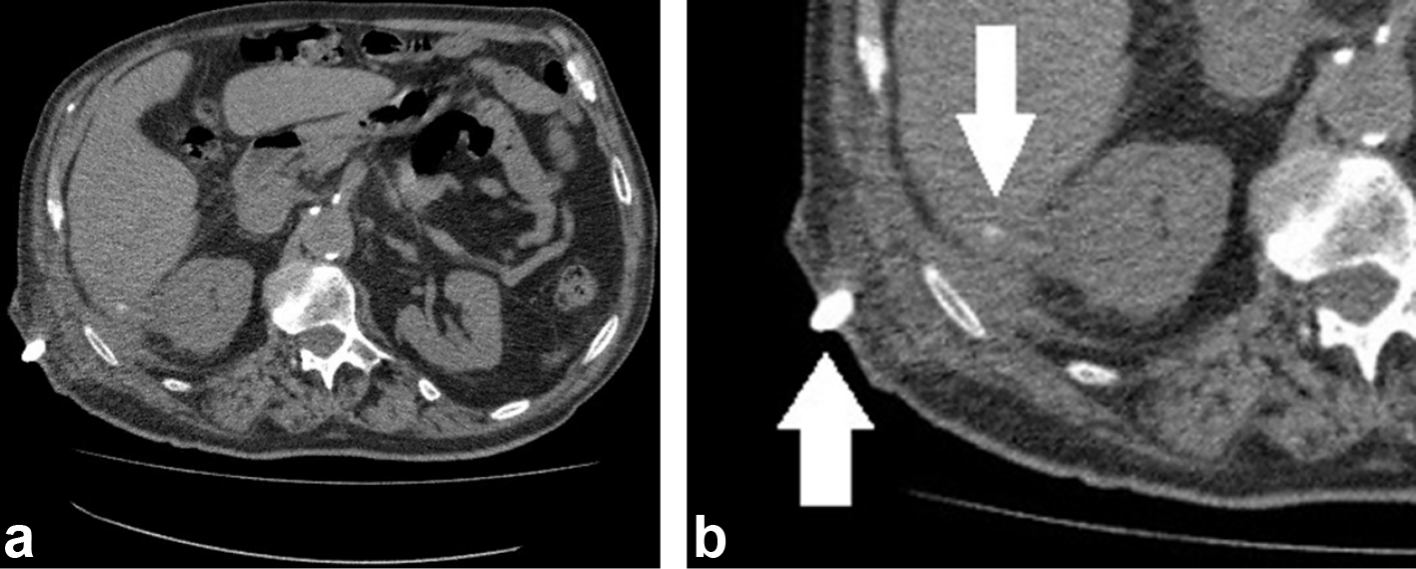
Unenhanced abdominal CT showing (a) partially resolved collection and (b)
